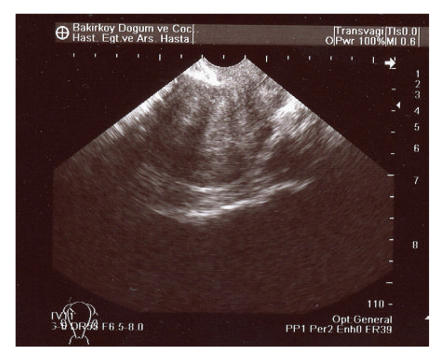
Transvaginal pelvic ultrasound showed an abnormal endometrial echo pattern. The endometrium was heterogenous and had irregular surface.
